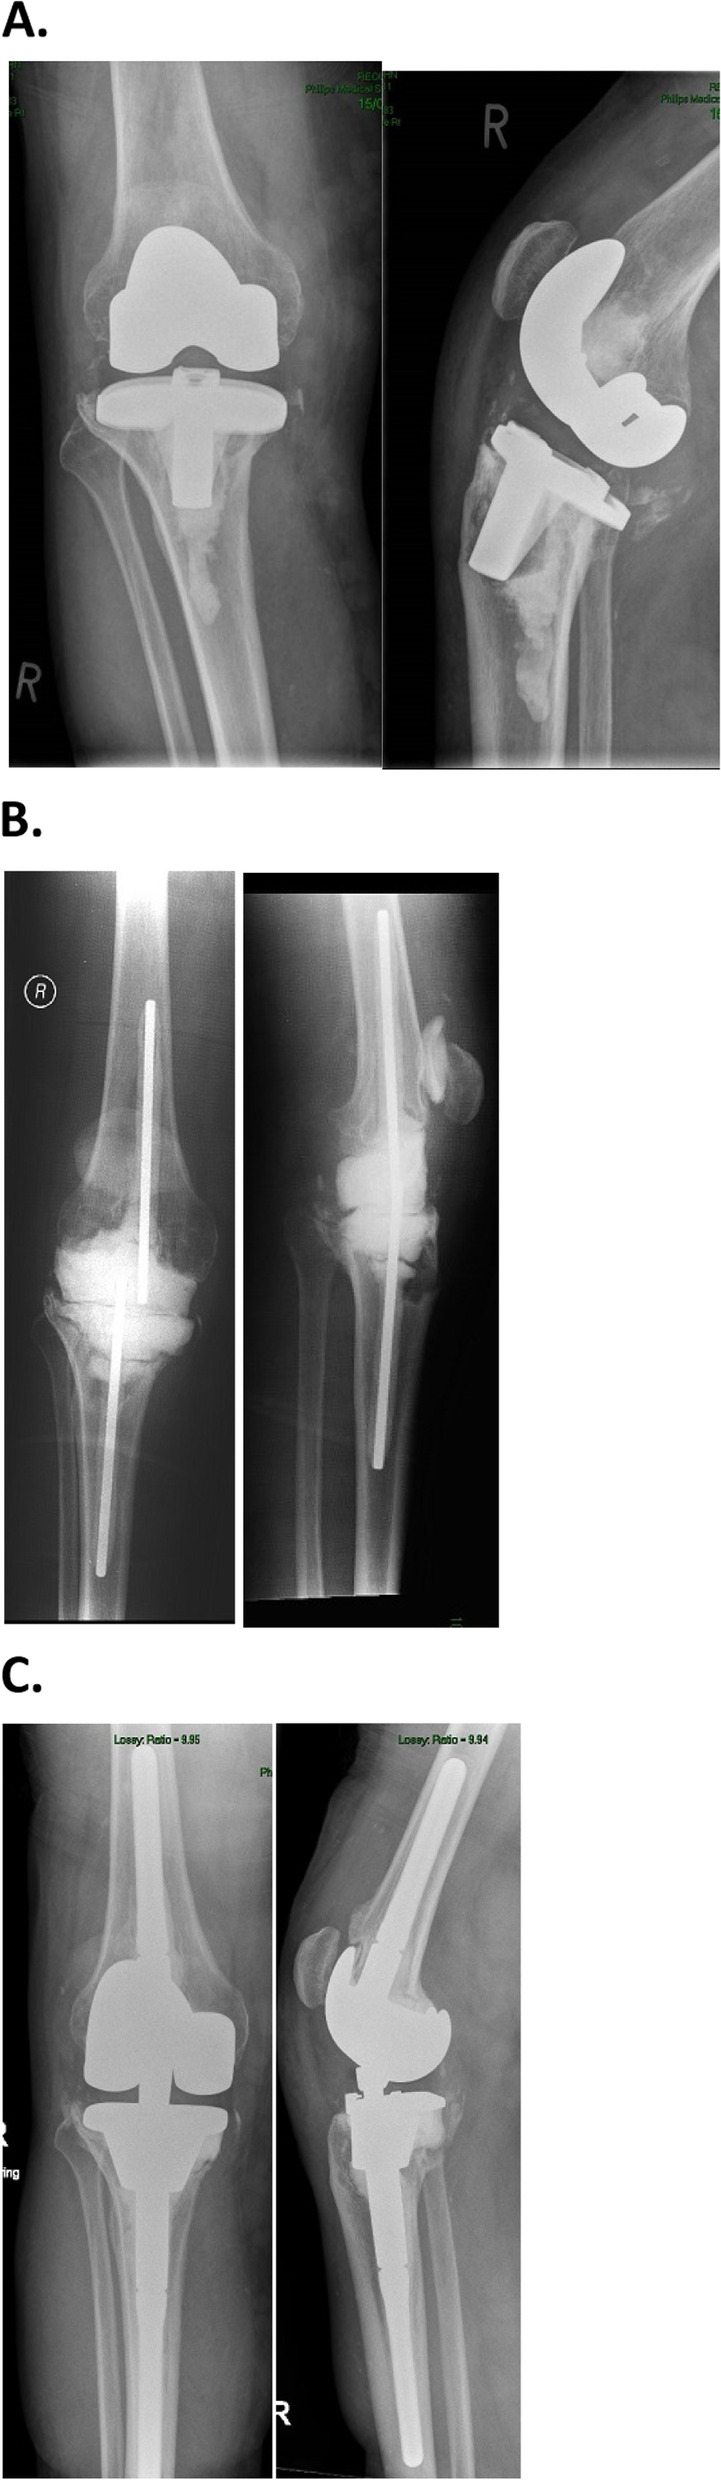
Radiographs (a-p and lateral view) of a surgical case, right knee TKA after low grade infection with coagulase-negative *Staphylococcus* 2 years post-op with catastrophic implant failure (a), after implant removal and 1st stage static antibiotic cement spacer armed with IM femoral and tibial rods (b), and 2nd stage revision with tibial metal cone and rotating hinge revision implant, 3 years post-op (c)
